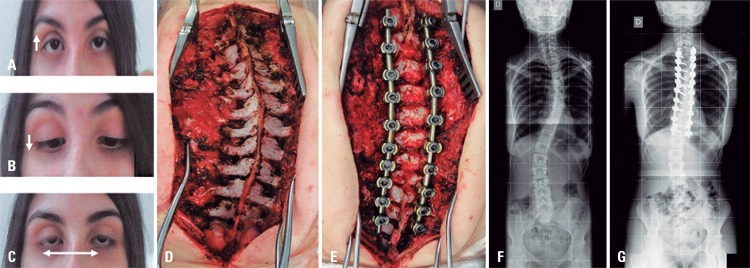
Operative findings. (A and B) Vertical eye movements within normal limits. (C) Absence of surgical features of horizontal eye movements. (D) Exposure of thoracic spine scoliosis with left convexity. (E) Posterior spinal fusion from the second to the eleventh thoracic level. (F) The anteroposterior radiograph before surgery. (G) The anteroposterior radiograph after surgery, showed degree of coronal balance and correction obtained
